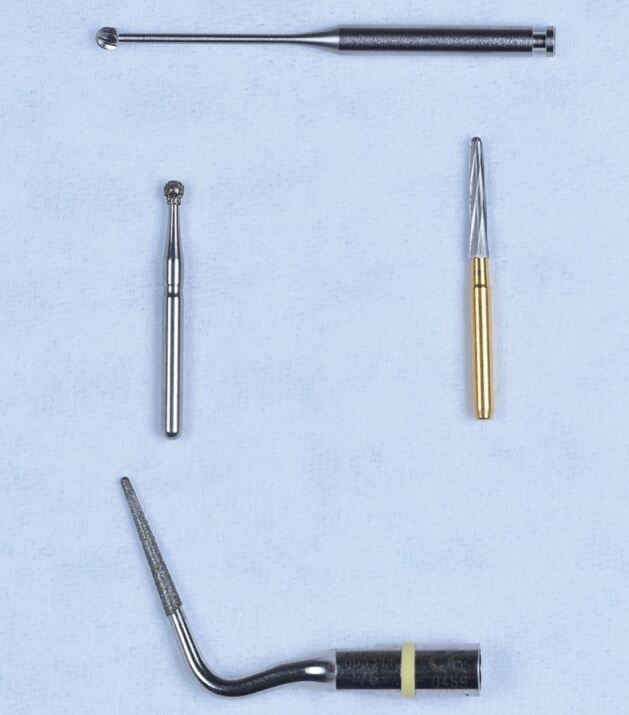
End-cutting round diamond bur, often used for endodontic access followed by a non-end-cutting tungsten carbide Endo-Z bur (Dentsply Sirona, USA), often used for deroofing of the pulp chamber. A Meisinger bur (Hager & Meisinger, Germany) is also shown and can be used for refining the access cavity and troughing to identify canal orifices (note the long shank which can aid operator visibility during access). A diamond-coated ET18D ultrasonic tip (Acteon, UK) is also shown and can be used for this purpose also
